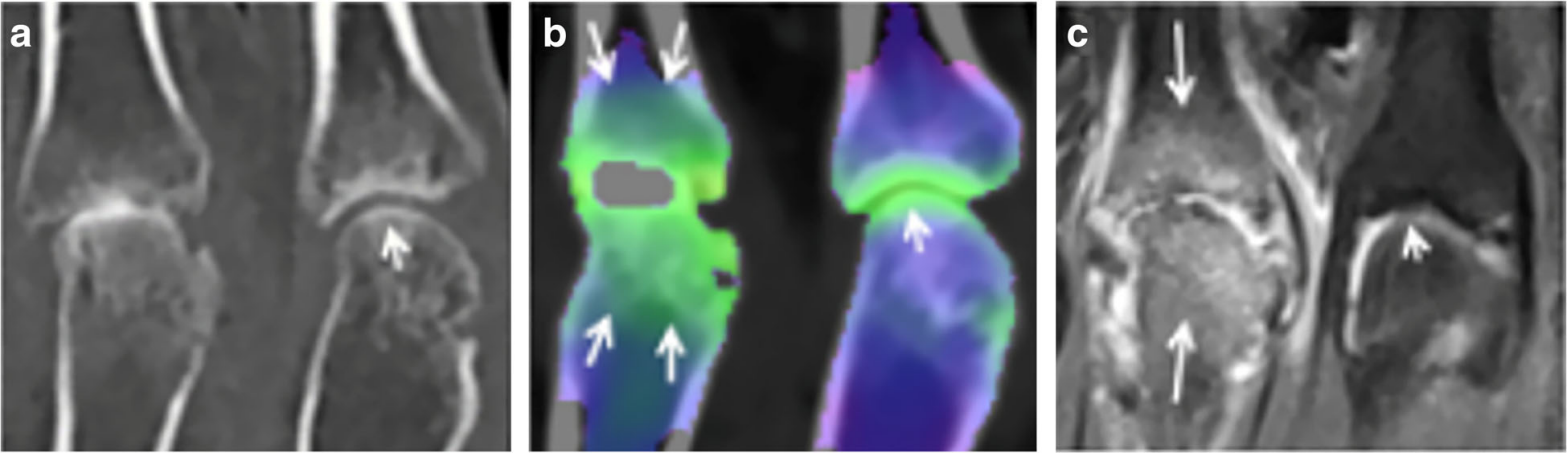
Dual-energy computed tomography scan of hands in a patient with rheumatoid arthritis. Magnification on the second metacarpophalangeal joint shows a normal and smooth outline of cartilage and bone plate (*short arrows*) on standard grayscale series (a) and normal virtual non-calcium attenuation (b). Conversely, bone marrow edema is evident on the third metacarpophalangeal joint presenting as an extensive and ill-defined green area (*long arrows*). Magnetic resonance imaging by means of T2-weighted fat-saturation sequence confirmed the presence of inflammatory bone marrow edema (c). From Jans et al. [72]
